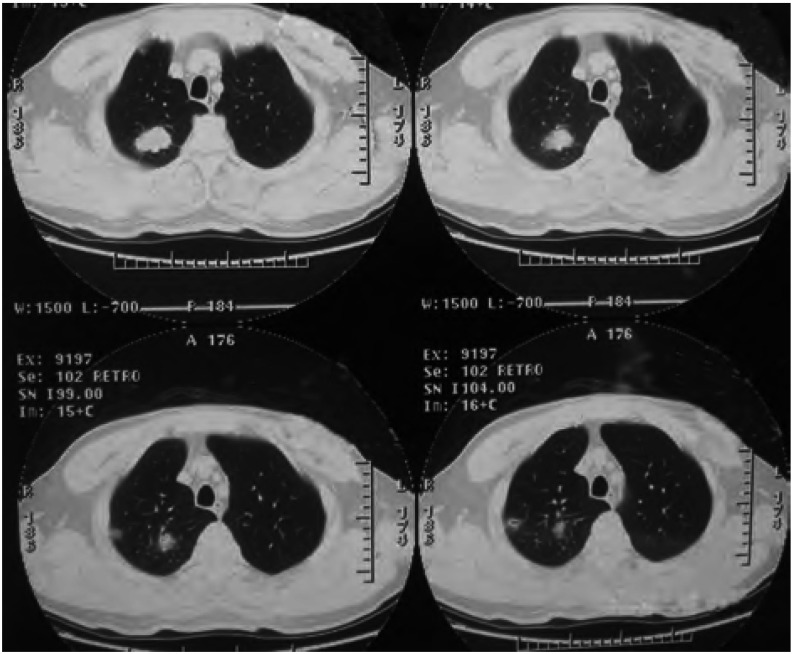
胸部CT。右肺上叶尖段可见3.3 cm×3.0 cm×2.6 cm软组织密度影，呈分叶状，周围可见毛刺；右肺上叶胸膜下可见一空洞性病变，直径约1.0 cm，其内壁不光滑，外壁毛糙。 Chest CT shows a lobulated parenchyma density shadow with glitch at the apical segment of the superior lobe of right lung, 3.3 cm ×3.0 cm×2.6 cm; another lesion has a cavity, the diameter of the cavity is 1.0 cm, with coarse intine and exine.
